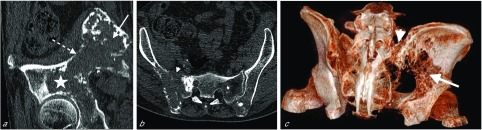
(a) Oblique sagittal reformatted view of pre-treatment CT (bone window, slice thickness 1 mm) of the iliac bone shows the extensive lesion involving the iliac wing (arrow), arcuate line (dotted arrow) and acetabulum (star). (b) Oblique axial reformatted view of pre-treatment CT (bone window, slice thickness 1 mm) of the pelvic bones shows the extensive lesion involving both the iliac bone and the sacral wing (arrowhead). (c) 3D oblique posterior reformatted view of the pelvic bones shows the extensive osteolytic lesion involving the iliac wing (arrow) and the sacral wing (arrowhead) greatly compromising pelvis stability.
